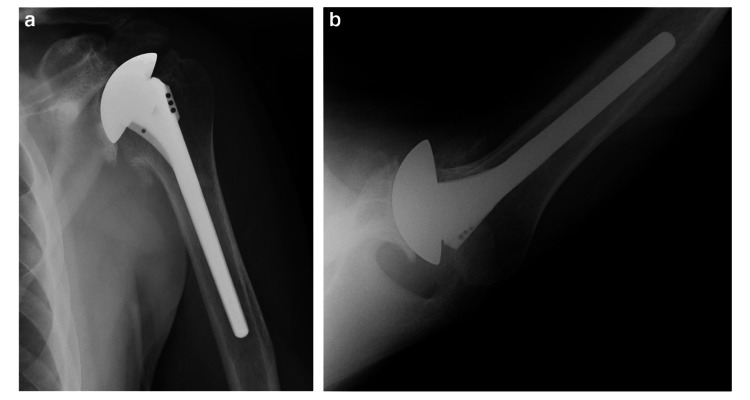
Postoperative Radiographs Following Ream-and-Run Humeral Hemiarthroplasty Postoperative anteroposterior (AP) and axillary radiographs showing centering of an anteriorly eccentric humeral head in the reamed glenoid, and fixation of a smooth-stemmed humeral component with impaction autografting. Used with permission from Matsen et al., International Orthopaedics (2019).
